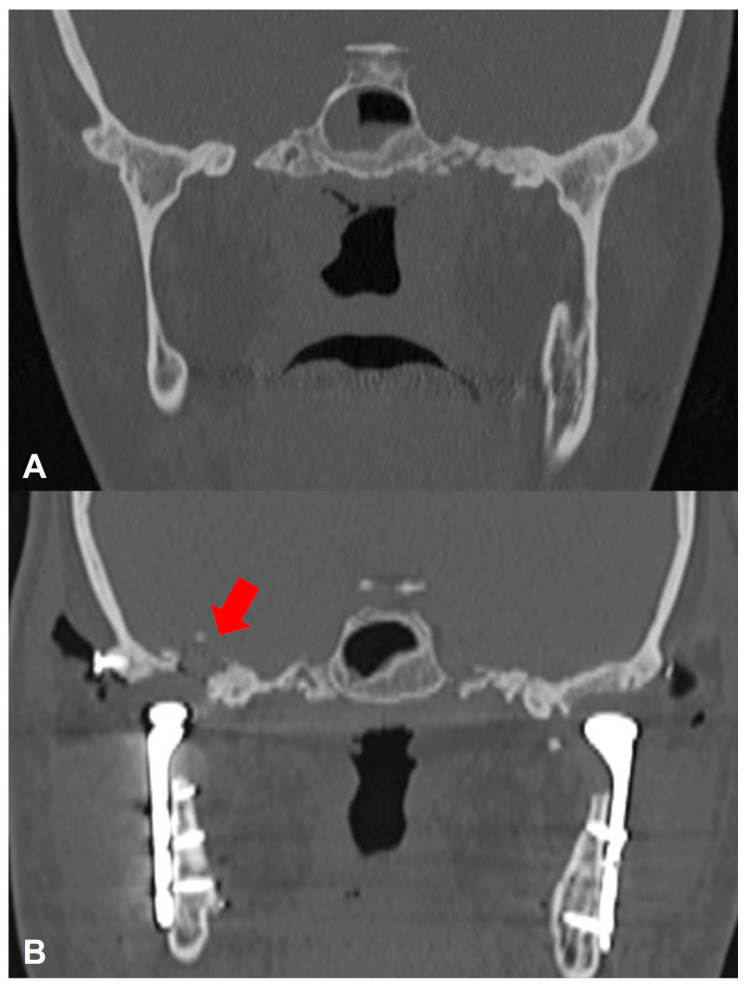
(A) Preoperative axial CT demonstrating bilateral full bony ankylosis. (B) Postoperative axial CT demonstrating skull base perforation at the right glenoid fossa (red arrow).
